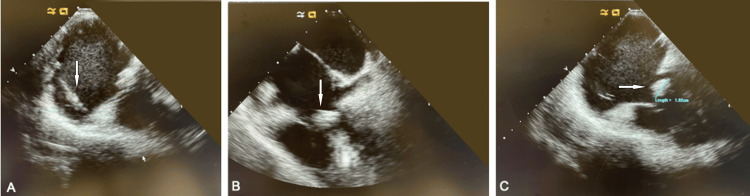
Transesophageal echography. (A) The pacing wire is visible in the right atrium. (B) The pacing wire is visible in the right ventricle. The vegetation is located across the tricuspid valve. (C) The vegetation measures 1.9 cm in length.
